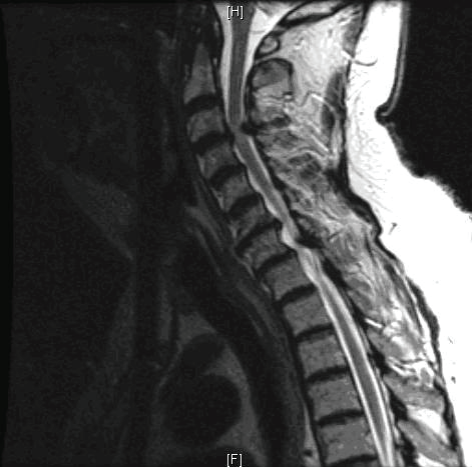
MRI T2 of the cervical spine showing multilevel spondylosis and moderate cord impingement at C3-C4.
